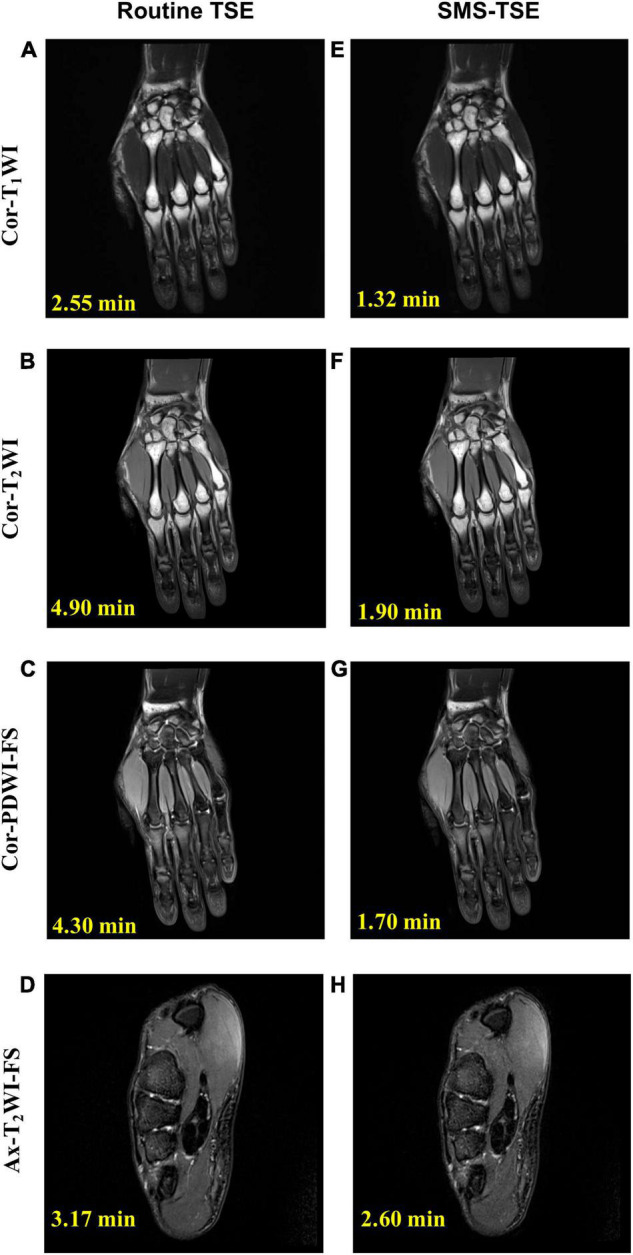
Comparison of high-resolution turbo spin-echo (TSE) sequence and simultaneous multi-slice-TSE sequence in the wrist joint imaging. The median nerve is thickened and swollen and shows hyperintensity on axial-T2-weighted imaging-fat saturation imaging (arrow), and the final diagnosis is carpal tunnel syndrome. All the images scored 5 in the image quality evaluation. For routine TSE, the SNR for Cor-T1WI (A), Cor-T2WI (B), Cor-PDWI-FS (C), and Ax-T2WI-FS (D) were 228.46, 108.76, 279.91, and 123.75; and the CNR for each was 192.21, 313.92, 72.77, and 71.55. For the SMS-TSE, the SNR for Cor-T1WI (E), Cor-T2WI (F), Cor-PDWI-FS (G), and Ax-T2WI-FS (H) were 208.36, 103.21, 182.86.91, and 103.71; and the CNR for each was 203.31, 308.65, 58.76, and 63.45.
